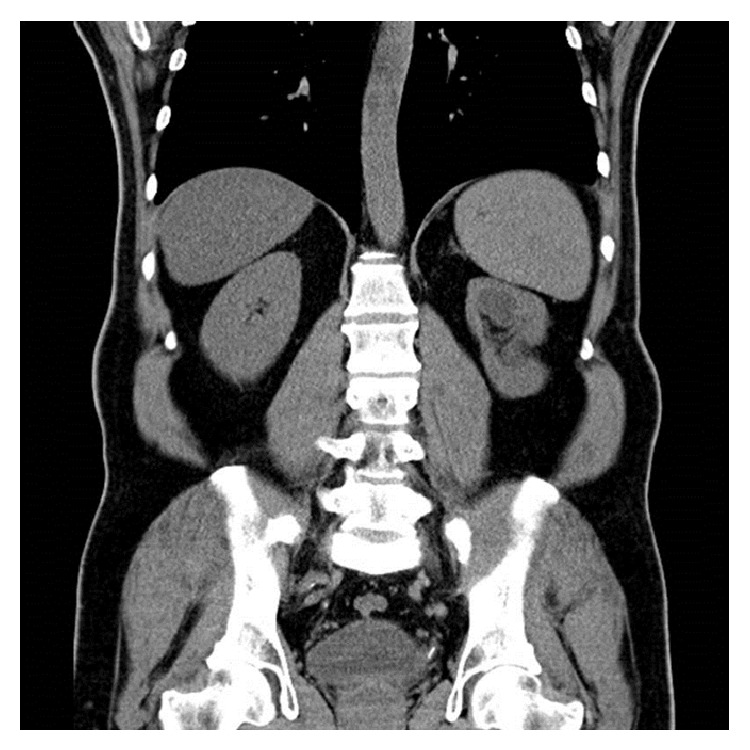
Postoperative CT image at the first month.
